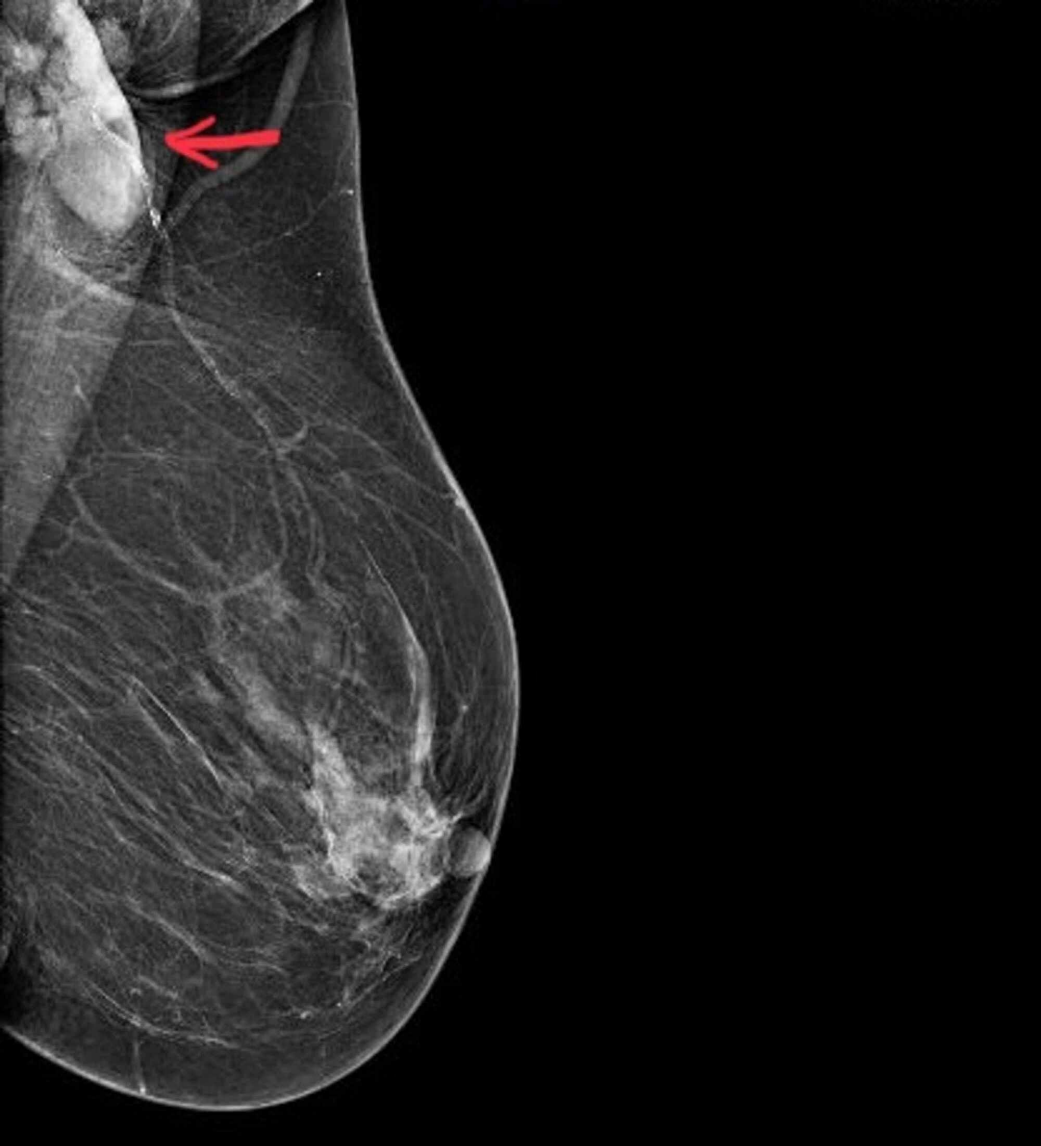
Mediolateral oblique view of left breast and axilla showing an equal to high-density oval lesion in the left axilla. Ultrasonography of the corresponding lesion showed a heterogenous hypoechoic finely spiculated lesion in the left axilla.
